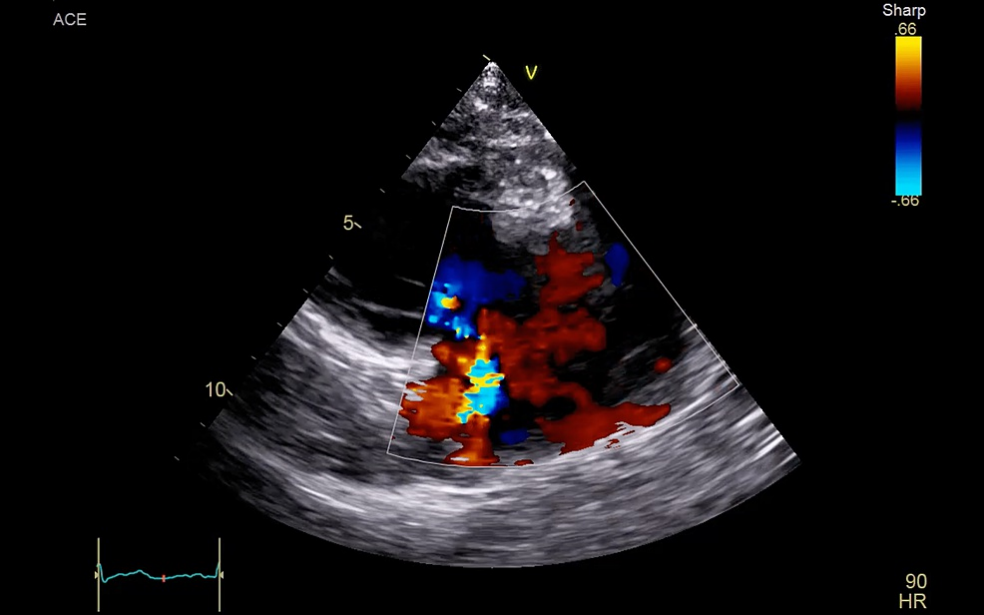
A right ventricular inflow view with color doppler of the first transthoracic echocardiogram showing tricuspid regurgitation.
